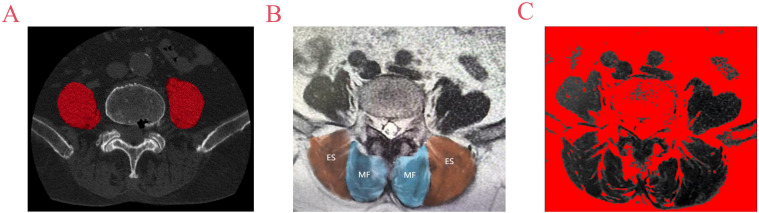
Imaging-based muscle measurements. (A) Manual segmentation of bilateral psoas muscles on axial CT at L4 (red) for CSA and PMI calculation. (B) ROI delineation of the paraspinal muscles on axial MRI at the inferior L4 endplate, including MF and ES. (C) Threshold-based segmentation in ImageJ to quantify FI; red mask indicates pixels meeting the preset threshold.
